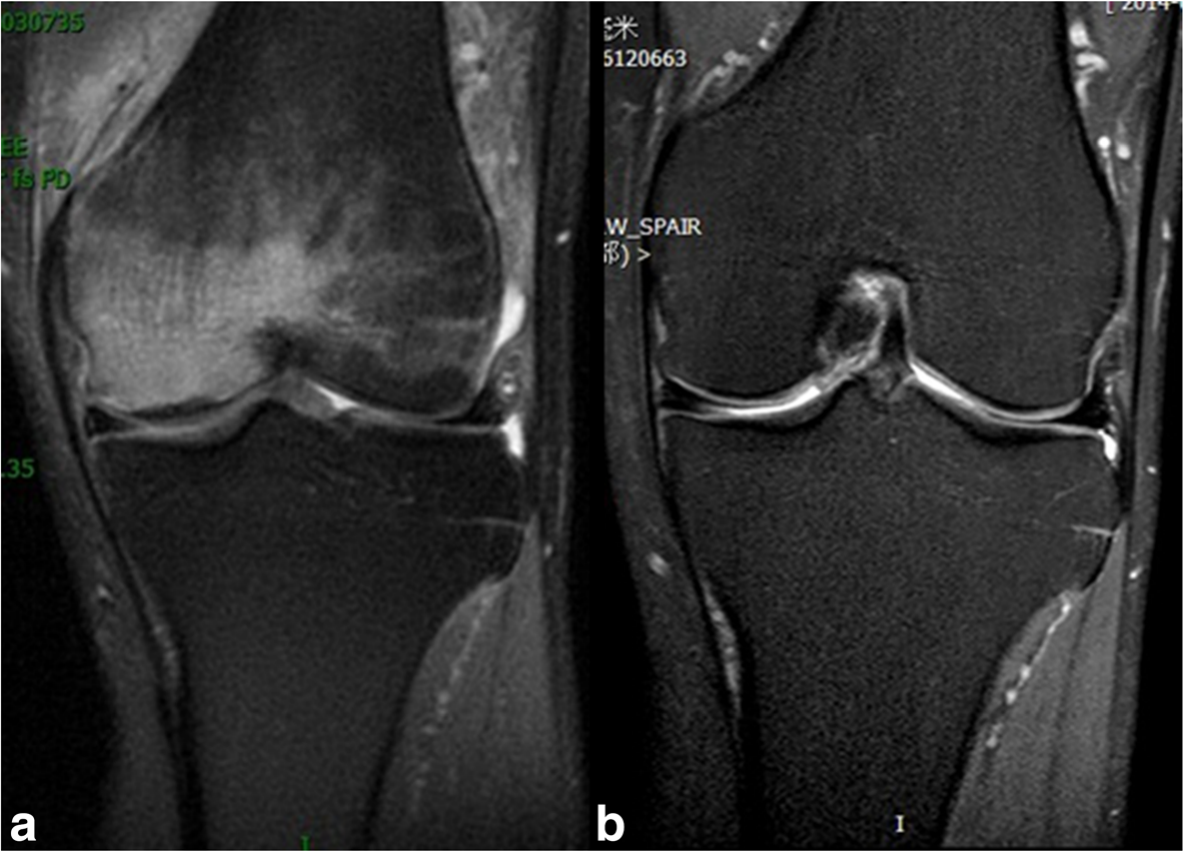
Pre- (a) and 6 months posttreatment (b) T2-weighted images showing the normalization of a large bone marrow edema within the medial femoral condyle of the left knee, in a 62-year old male patient. (Note: The patient consented to publish the specific information.)
